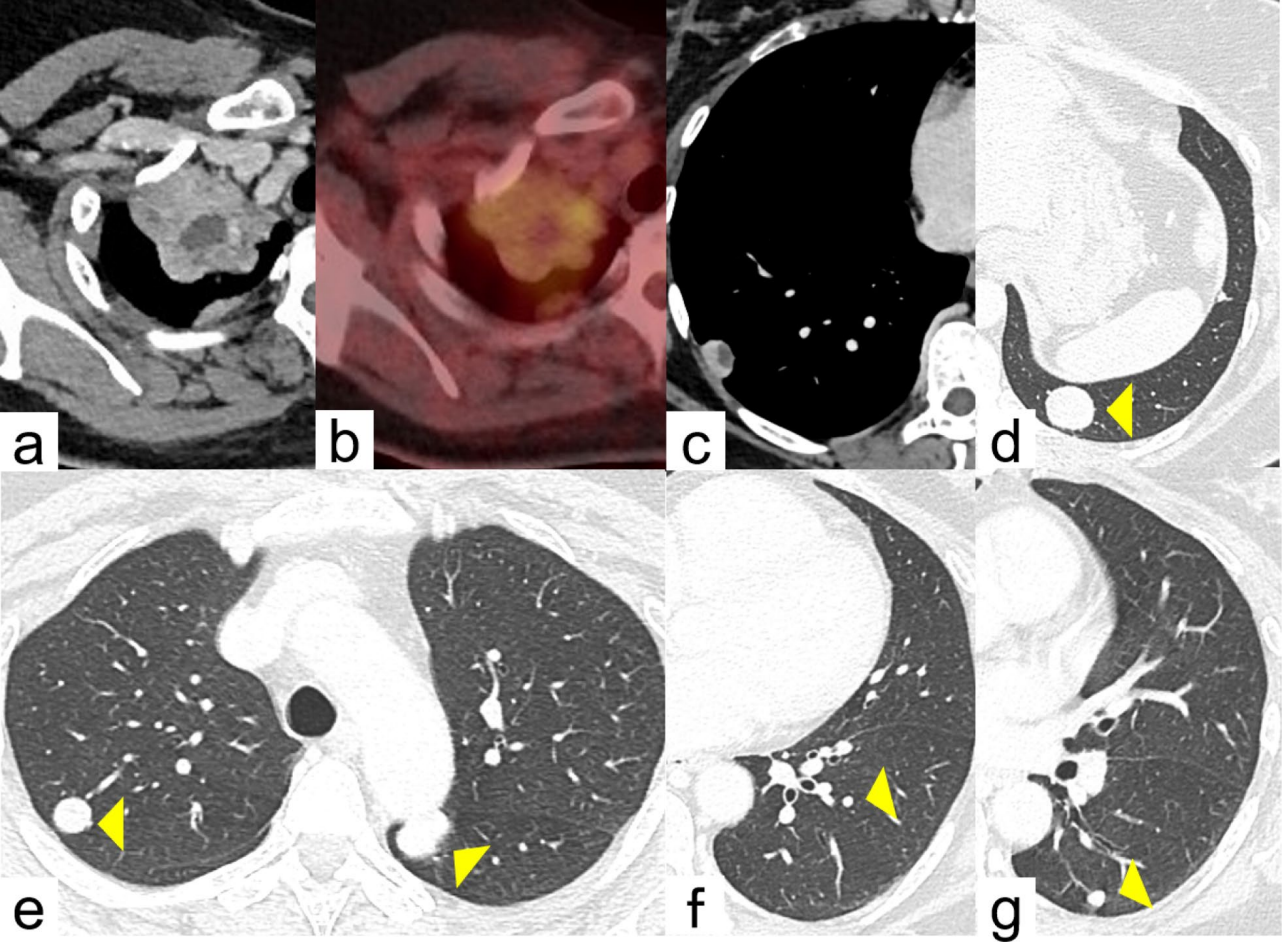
Computed tomography and positron emission tomography findings before the left lung biopsy. a The right upper lobe lesion contained a cystic fluid component. b Positron emission tomography revealed moderate avidity (maximum standardized uptake value, 4.93) in the right upper lobe lesion. c The tumor in the right lateral basal segment contained a cystic fluid component. d–g Solid tumors were located in the bilateral lungs (yellow arrowhead)
